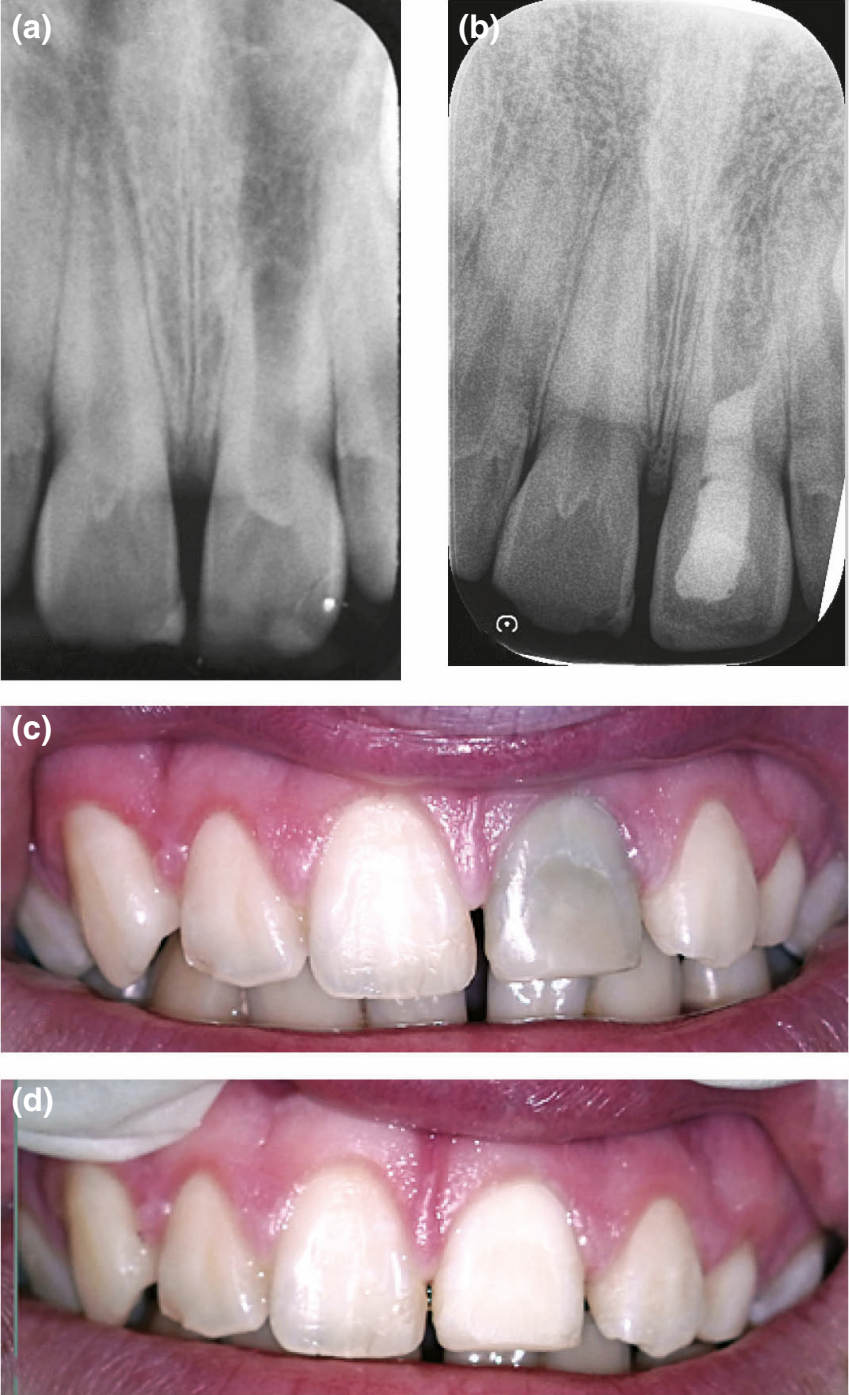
(a) A preoperative radiograph showing a left maxillary central incisor with interrupted root development indicating pulp necrosis. (b) The tooth as treated with regenerative endodontic protocols. (c) A photograph revealing blackish/greyish discolouration. (d) A photograph of the tooth after three bleaching appointments using the protocol described in Figure 5. Reprinted in part from (Fida et al., 2021) with permission
